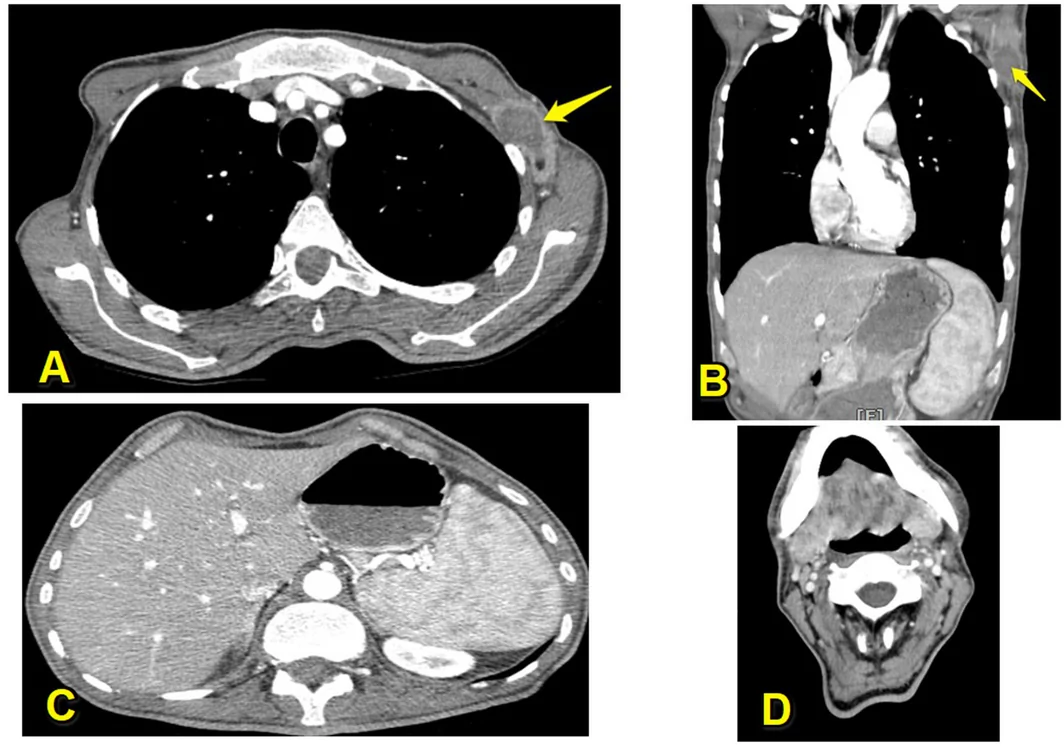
Contrast‐enhanced computed tomography (CT) findings obtained during the initial diagnostic evaluation. (A) Axial contrast‐enhanced chest CT demonstrates focal soft‐tissue thickening along the left chest wall (arrow). (B) Coronal reconstructed chest CT shows longitudinal extension of the chest wall lesion (arrow) without evidence of pulmonary parenchymal involvement. (C) Axial contrast‐enhanced abdominal CT reveals splenomegaly without focal hepatic or splenic lesions. (D) Axial contrast‐enhanced neck CT demonstrates no discrete cervical mass or pathological cervical lymphadenopathy. Collectively, these imaging findings suggested a systemic lymphoproliferative process and prompted further diagnostic evaluation, including excisional biopsy of the left axillary lymph node, which established the diagnosis of sporadic Burkitt's lymphoma.
